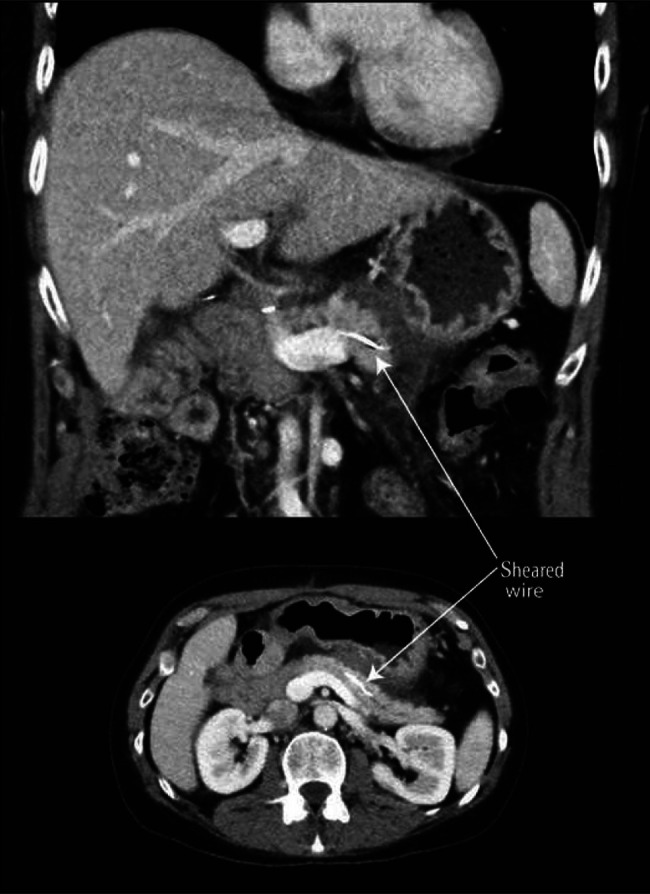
Computed tomography scan (coronal and axial views) identified the sheared wire within the lumen of the main pancreas duct.
